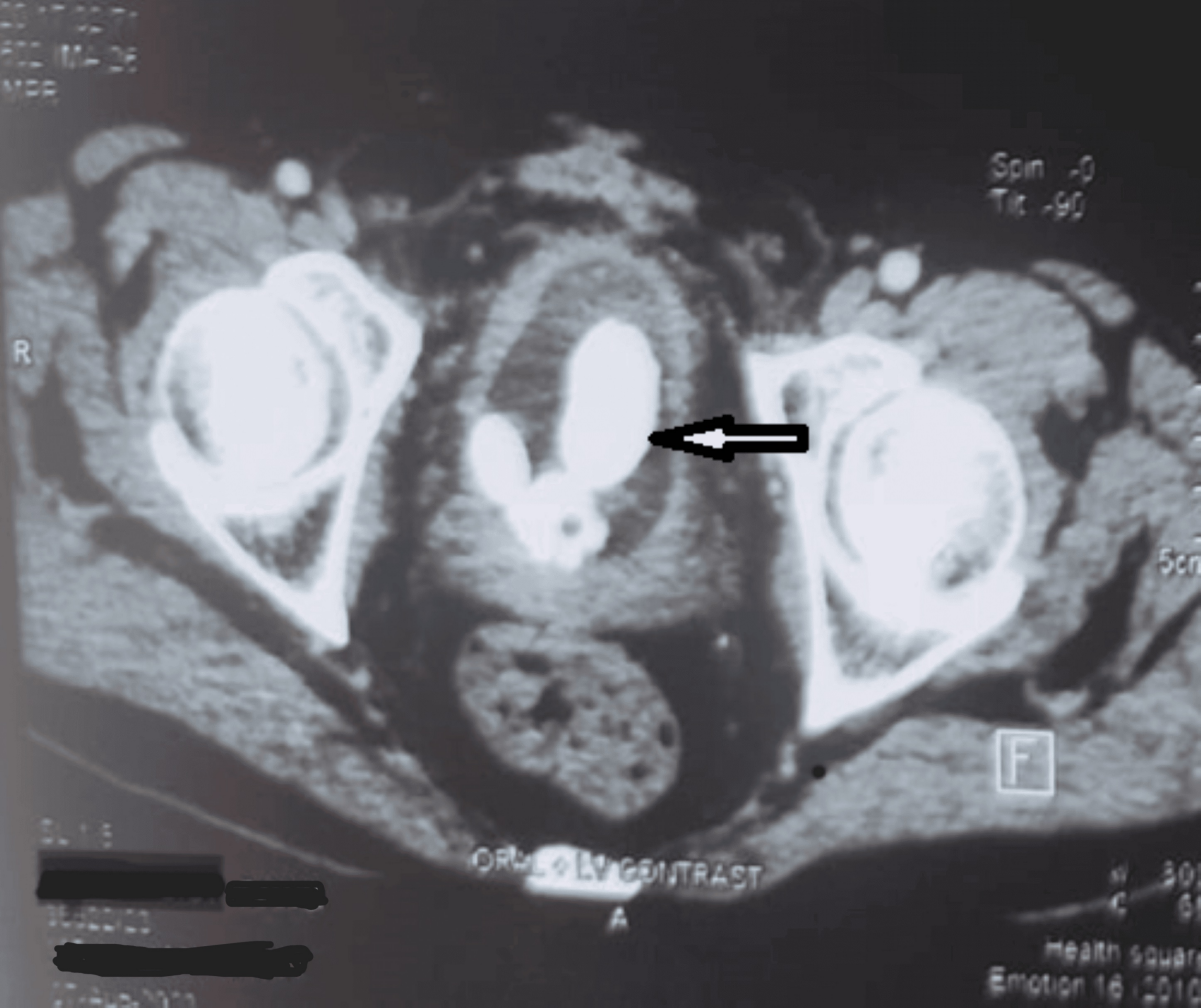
CT film showing intravesical multiple calculi
